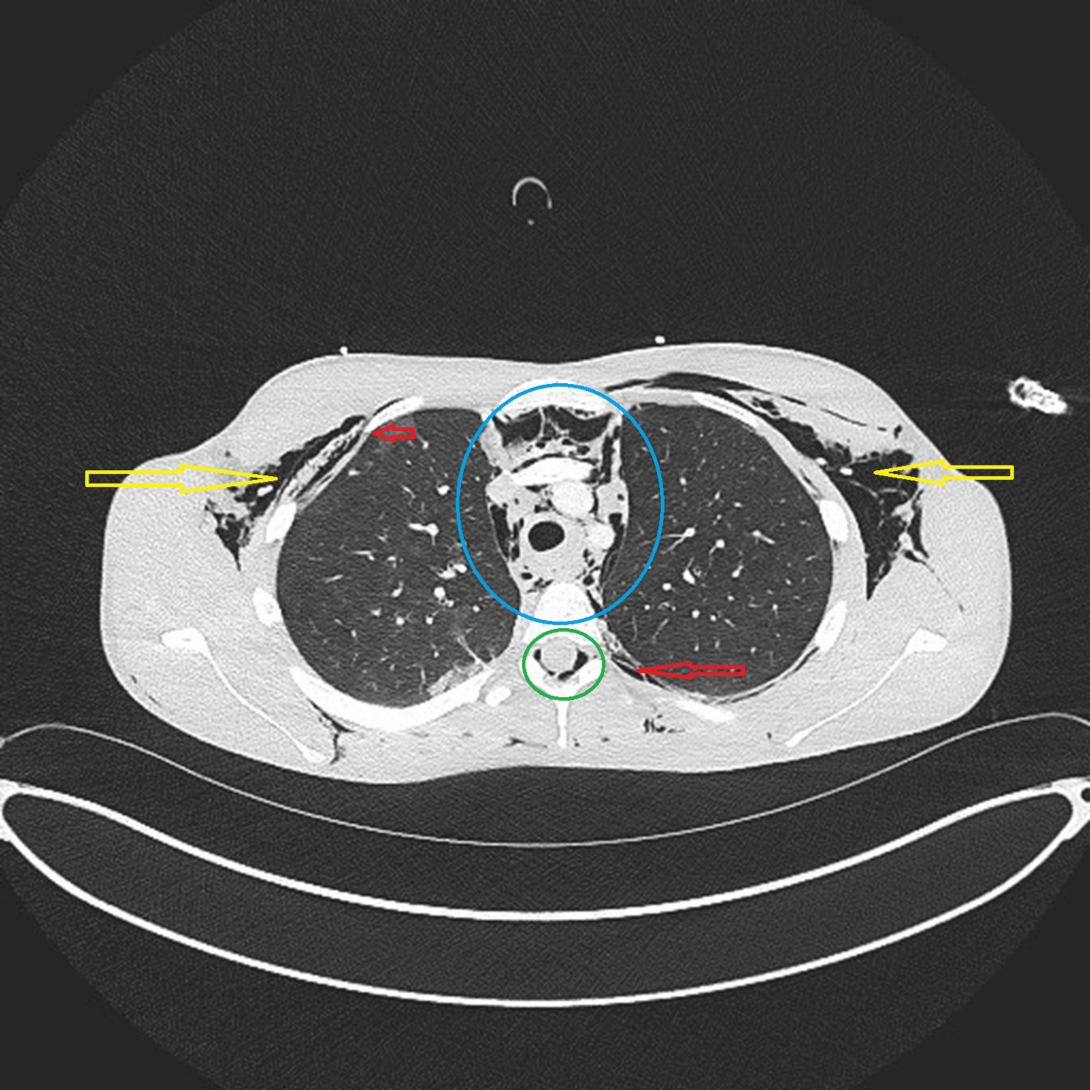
Contrast-enhanced CT chest Axial CT image demonstrating extensive subcutaneous emphysema (yellow arrows) and pneumomediastinum (blue circle). Pneumorrhachis is visible (green circle), and pneumothorax is highlighted (red arrows).
